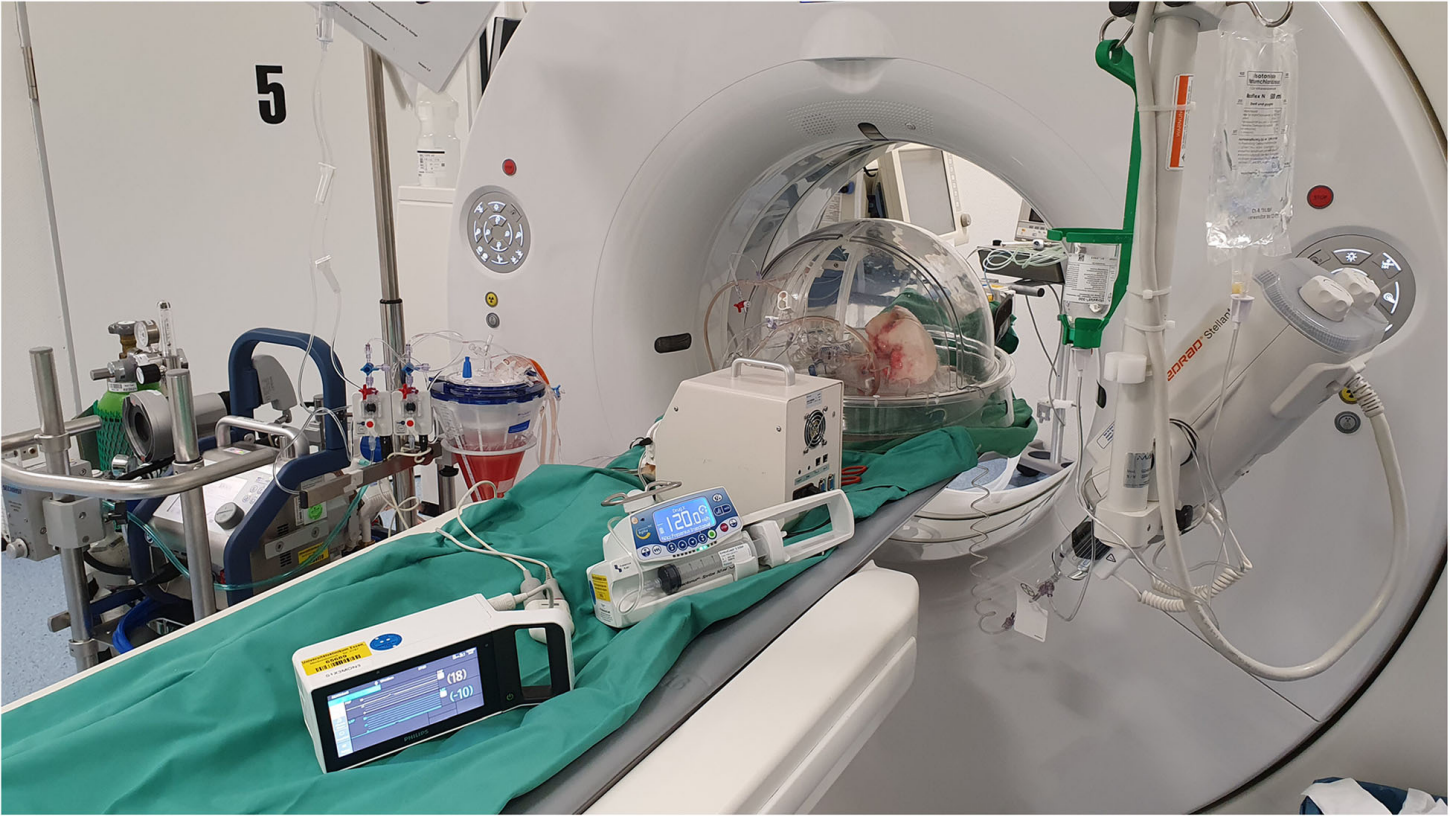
Actual setup. The lower lung lobe is positioned in the computed tomography gantry
